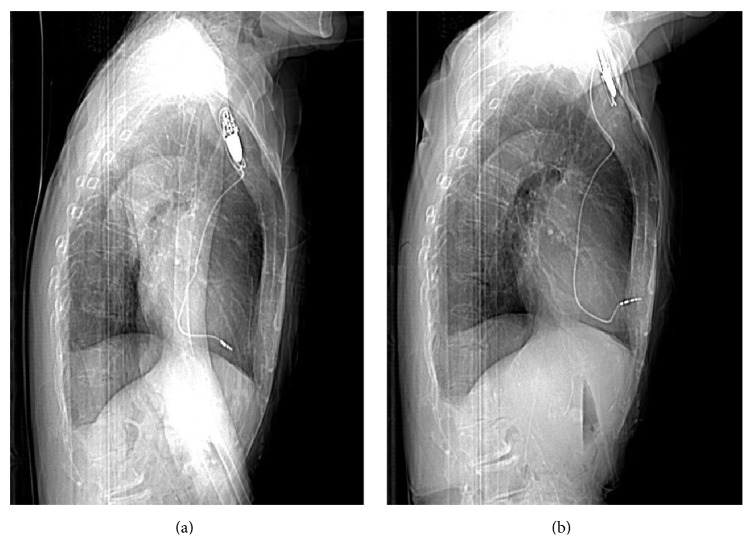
Lateral chest X-ray film demonstrating the position of the lead. (a) Just after the surgery. The tip of the lead was in the correct position. (b) Four days after the surgery. The tip of the lead intruded into the chest wall.
